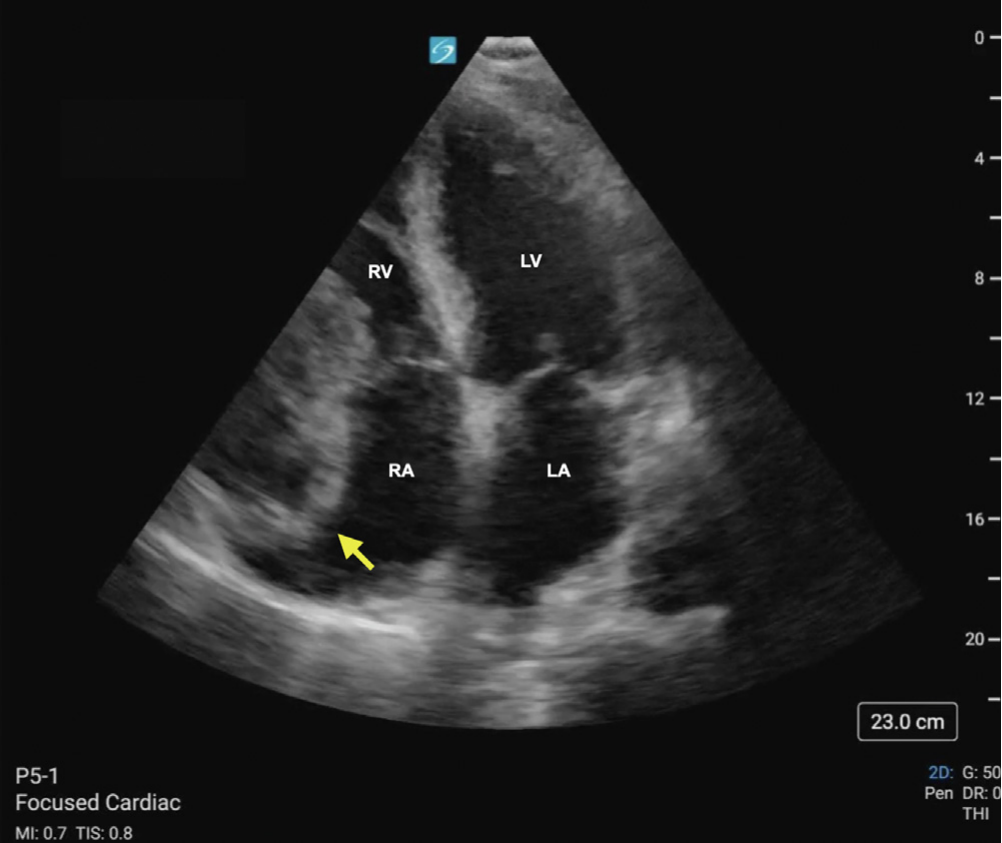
Still image of apical 4-chamber view of the heart with labels denoting cardiac chambers. The arrow denotes the right heart mass compressing the right atrium (RA) and right ventricle (RV). LA, left atrium; LV, left ventricle.
